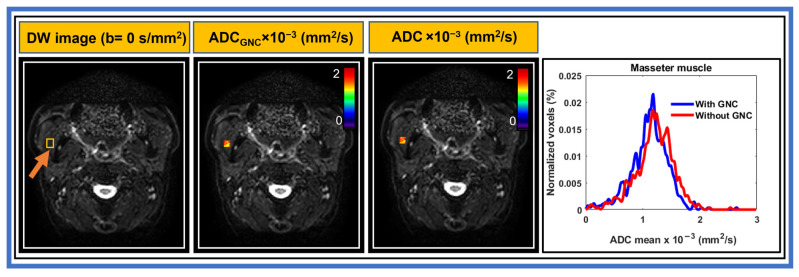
Example of diffusion-weighted MR images (b = 0 s/mm^2^) acquired using multi-shot echo planar imaging (EPI) and apparent diffusion coefficient (ADC) maps of masseter muscle (rectangle in a yellow color) with and without gradient nonlinearity correction (GNC) from a representative head and neck cancer patient (55-year-old male). The ADC maps exhibiting slightly lower ADC values after GNC. The histograms were of similar width and with minimal shift.
